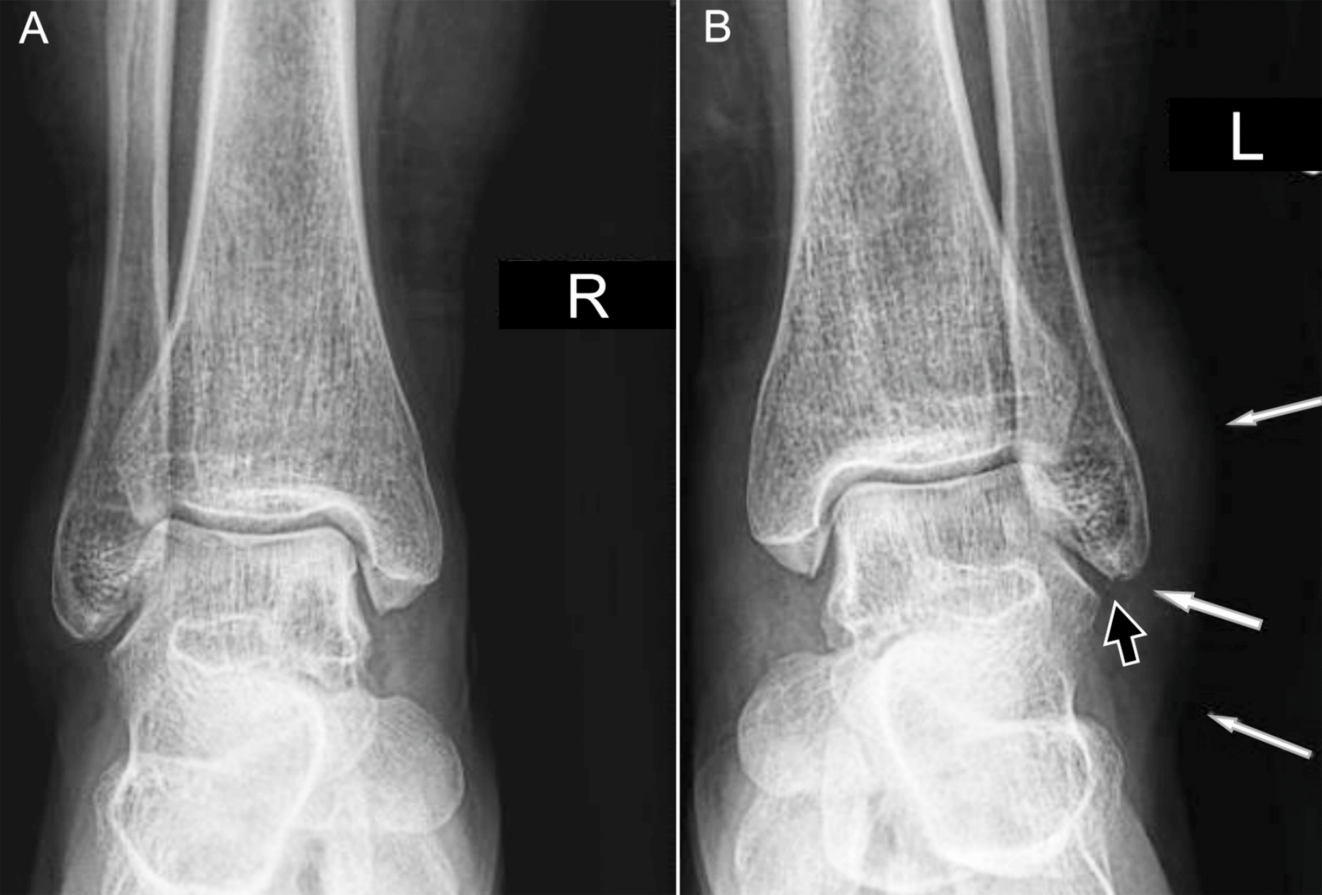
Comparative ankle radiographs after trauma. A 60-year-old female: (A) anteroposterior radiograph of the right ankle shows normal alignment and soft tissues; (B) anteroposterior radiograph of the left ankle shows cortical discontinuity at the fibular apex (black arrow) with lateral soft-tissue swelling (white arrows). Images belong to the authors; patient consent was obtained.
